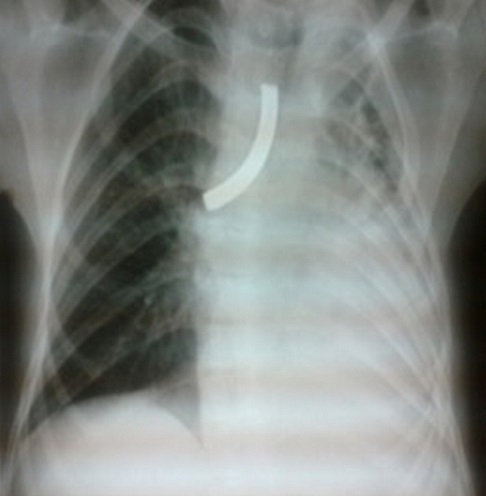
Radiographie thoracique montrant la canule en intra trachéal se dirigeant vers la bronche souche droite
